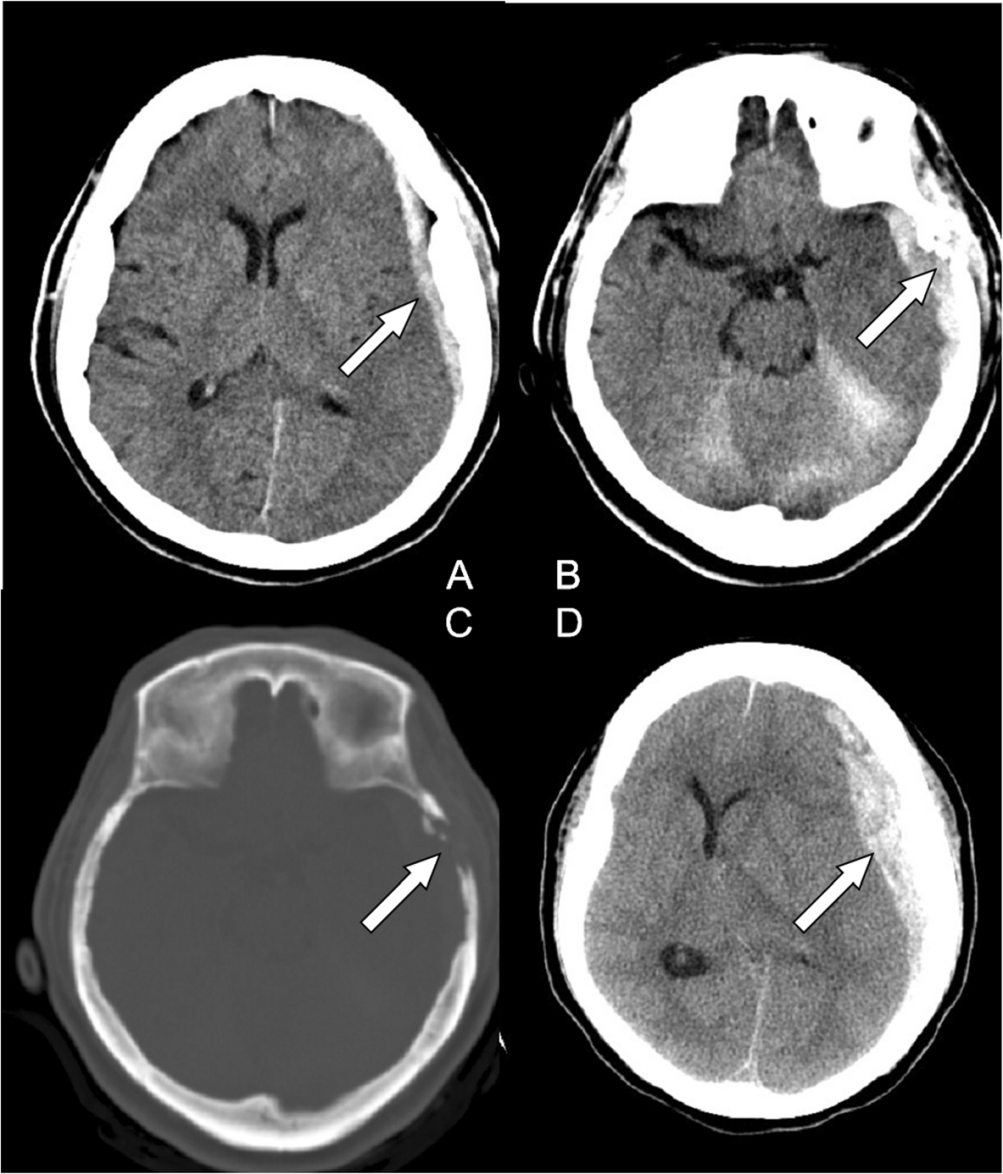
(a) Computed tomography (CT) of the head showing left temporal subdural hematoma (SDH) (arrow) with slight mass effect. (b) small left temporal bone erosive lesion (arrow). (c) A bony defect on the left temporal bone (bone window of CT) (arrow). (d) Enlargement of left SDH with significant mass effect (arrow)
